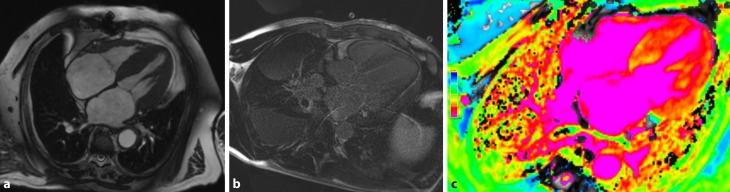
Cardiac MRI features in amyloidosis. The cine imaging shows hypertrophic left and right ventricles as well as severely dilated atria (a). Typical late enhancement pattern 15 min after gadolinium application (b). Native T1 mapping, which is used for extracellular volume quantification (native T1 time myocardium: 1180 ms; extracellular volume: 56.8%; c). Courtesy of Franz Ducka, MD (Medical University Vienna, Department of Internal Medicine II, Division of Cardiology)
